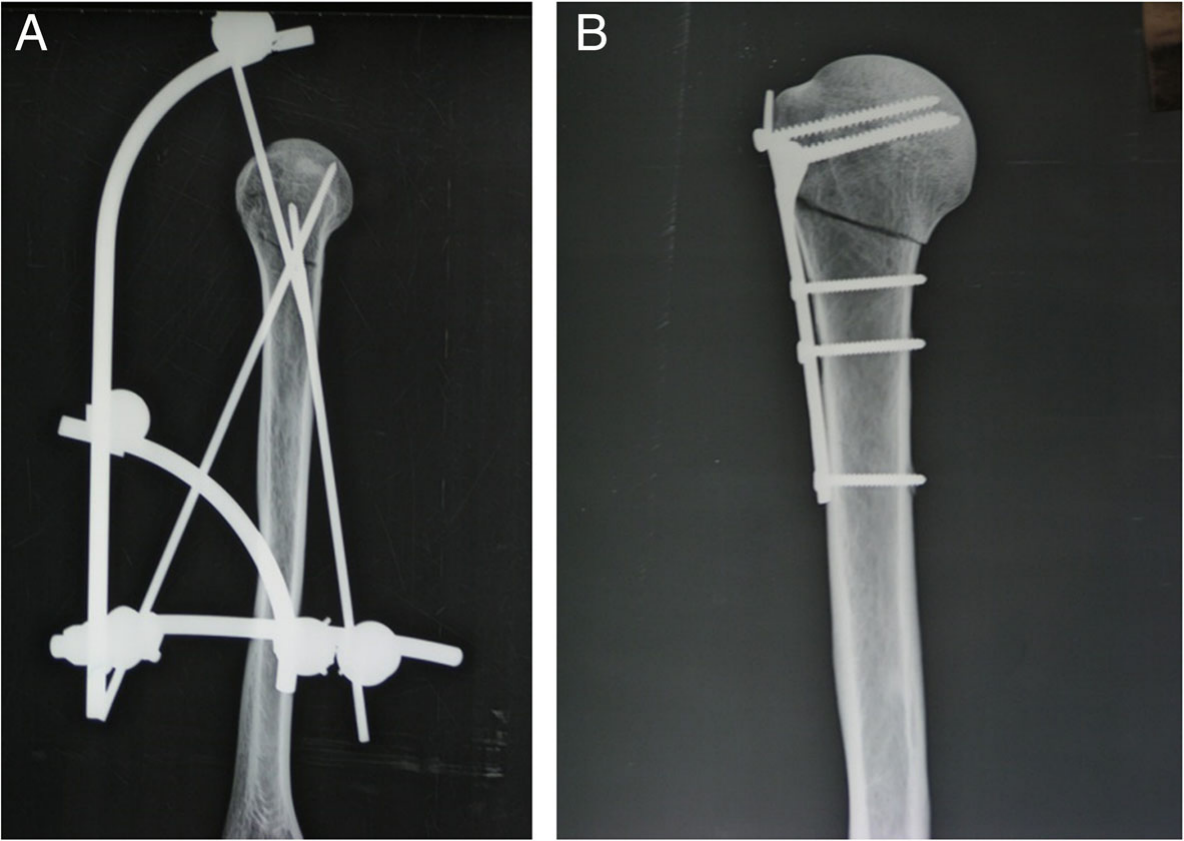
Lateral X-ray images of the external fixator (a) and plate fixation (b)
